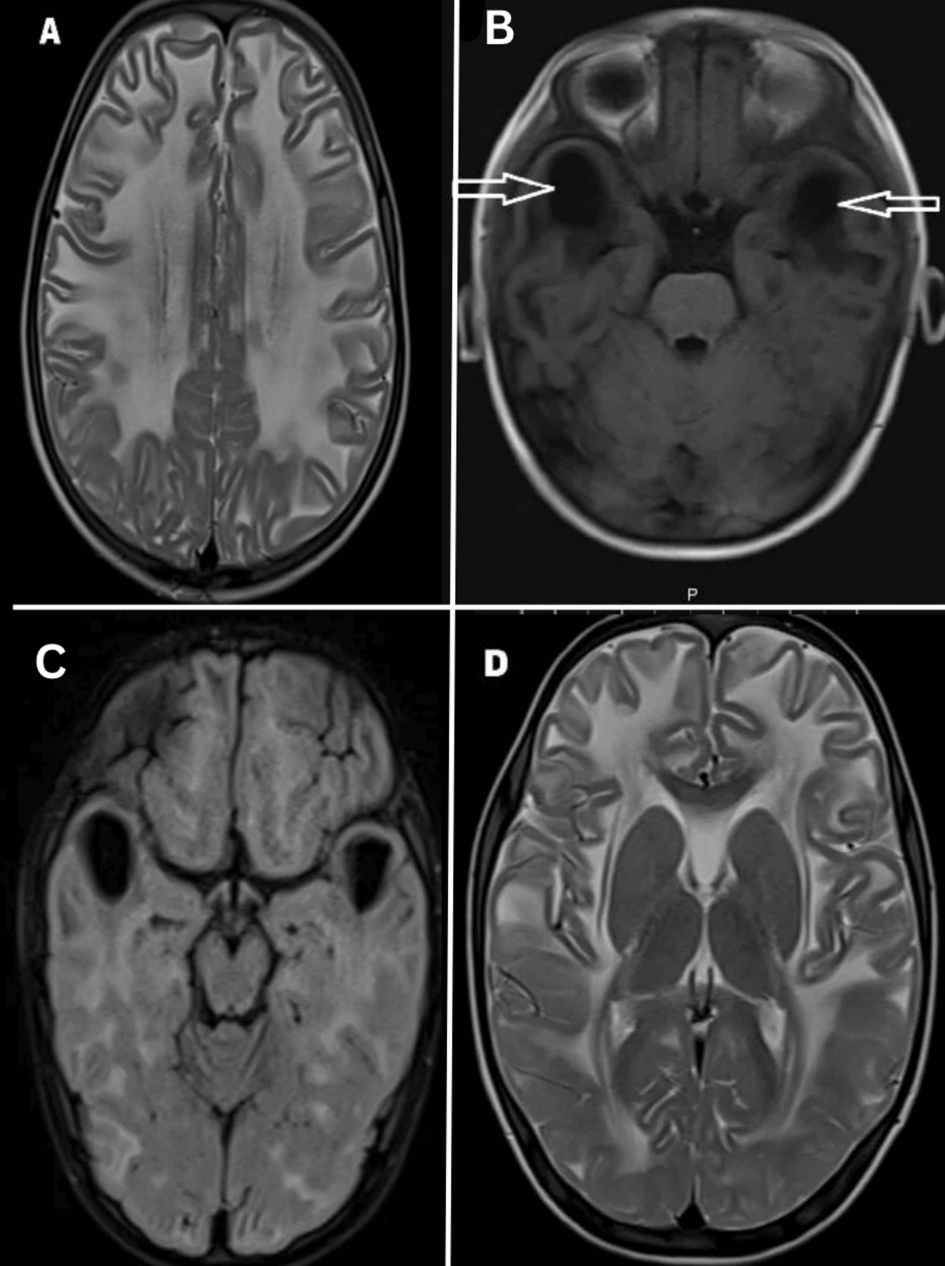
Brain MRI T2 WI (A) shows symmetrical diffuse bilateral hyperintensity (dysmyelination) involving subcortical and deep white matter of cerebral hemispheres along with formation of subcortical cysts bilaterally in the frontotemporal lobe as seen in T1 WI (B) and T2 fluid-attenuated inversion recovery (FLAIR) (C) sequence. B/L basal ganglia and B/L thalamus are spared as seen in T2 WI (D) WI: weighted imaging
